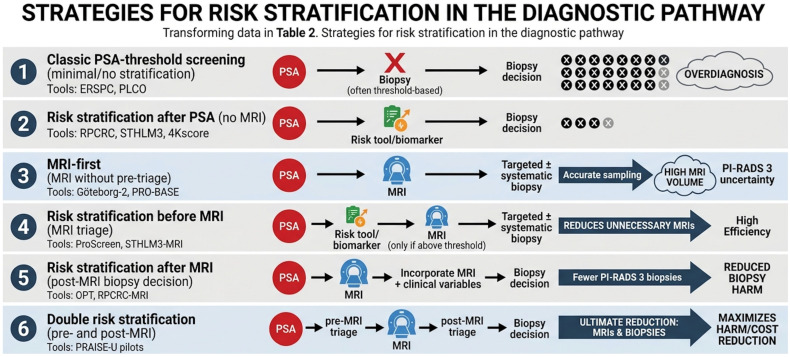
Strategies for risk stratification in the diagnostic pathway. PSA = Prostate-Specific Antigen; PCa = prostate cancer; csPCa = clinically significant prostate cancer; MRI = Magnetic Resonance Imaging;; RPCRC = Rotterdam Prostate Cancer Risk Calculator; STHLM3 = Stockholm3 model; ERSPC = European Randomized Study of Screening for Prostate Cancer; PLCO = Prostate, Lung, Colorectal, and Ovarian (Cancer Screening Trial); OPT = “optimal”/optimized post-test strategy (as used in some pathway descriptions; often referencing concepts like PSA density); PROBASE = Prostate Cancer Screening in Young Men (PROBASE trial); PRAISE-U = PRAISE-U pilots/trials (double risk-stratification pathway).
